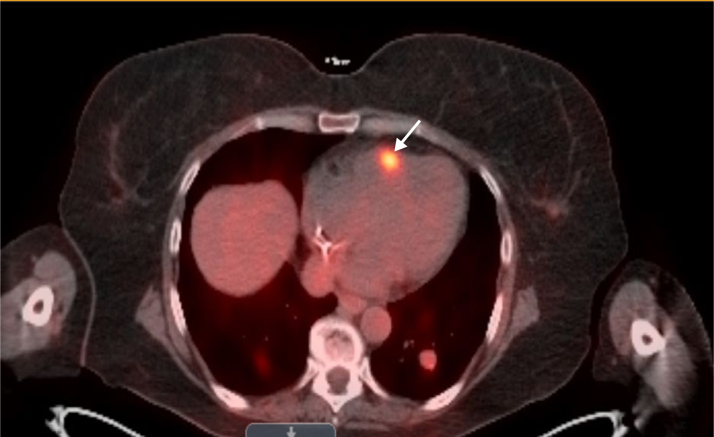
PET-CT Showing Multiple Nodular Cardiac Regions of Increased Metabolic Activity The arrow is pointing to the right ventricular mass. PET-CT = positron emission tomography–computed tomography.
